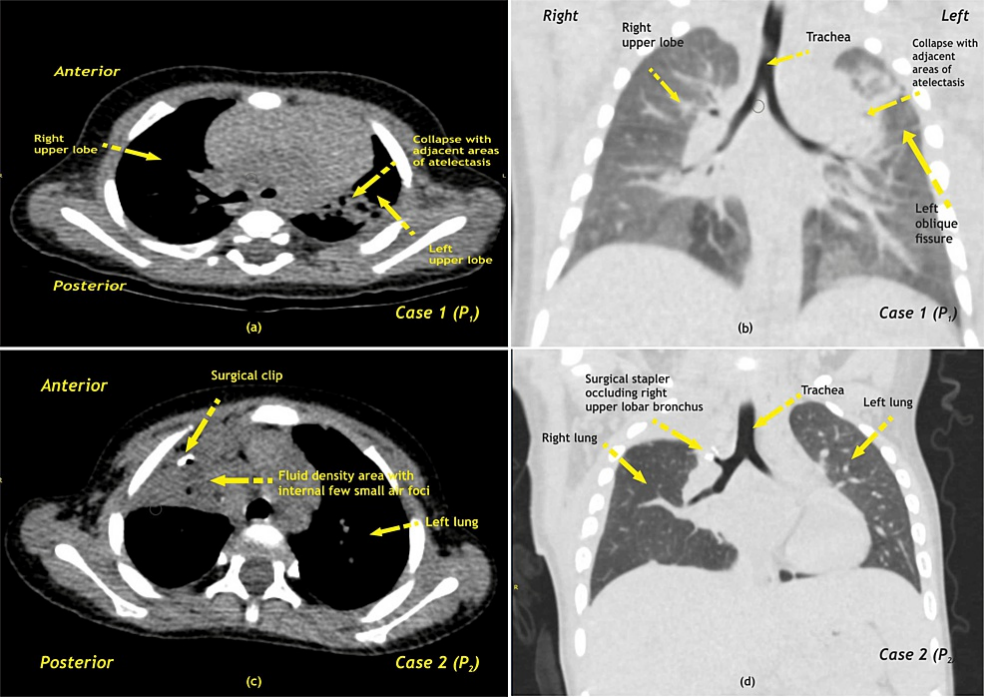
Case 1 (a) HRCT Axial scan chest in mediastinal window, (b) Coronal HRCT scan chest in lung window showing areas of collapse with atelectasis noted in apico-posterior and anterior segments of left upper lobe with mild volume loss of left upper lobe with mild upward displacement of left oblique fissure. Case 2 (c) Postoperative axial HRCT scan of chest in mediastinal window showing mild fluid density areas with internal few small air foci noted in apical and anterior area adjacent to first and second intercostal space right side. There are few internal radiopaque surgical clips noted, (d) Postoperative coronal HRCT scan of chest in lung window showing stapler occluding right upper lobar bronchus. There are areas of adjacent sub-segmental collapse noted. HRCT - High-resolution computed tomography; P - Patient Image Credit: Dr. Dharmendra B. Parmar
